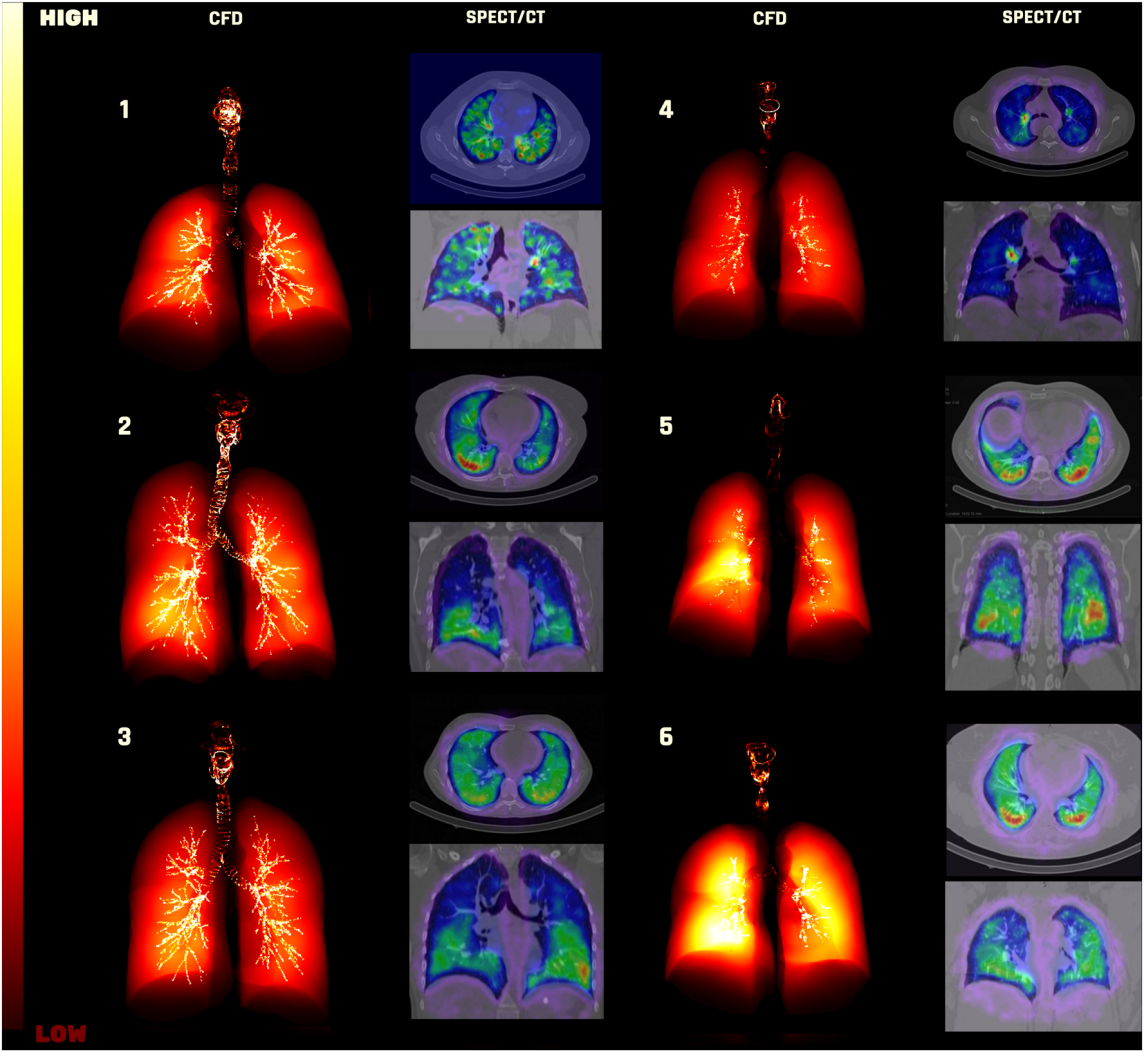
Qualitative comparison of between the predicted deposition and the experimental SPECT data presented in 15. For each patient, the figure on the left is the CFD results and the one on the right is obtained from SPECT.
